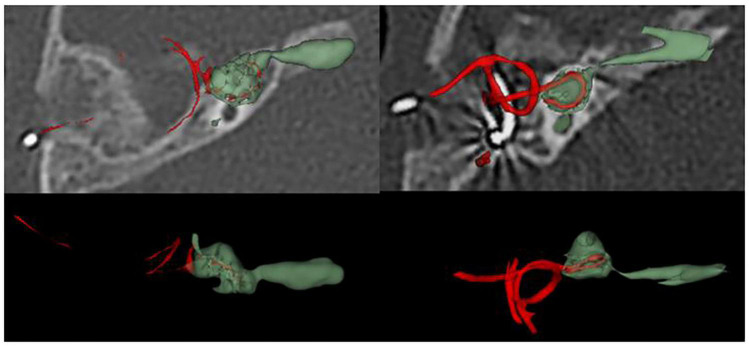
Computed tomography (CT) reconstruction of surgical side in patients with common cavity deformity (CCD).
